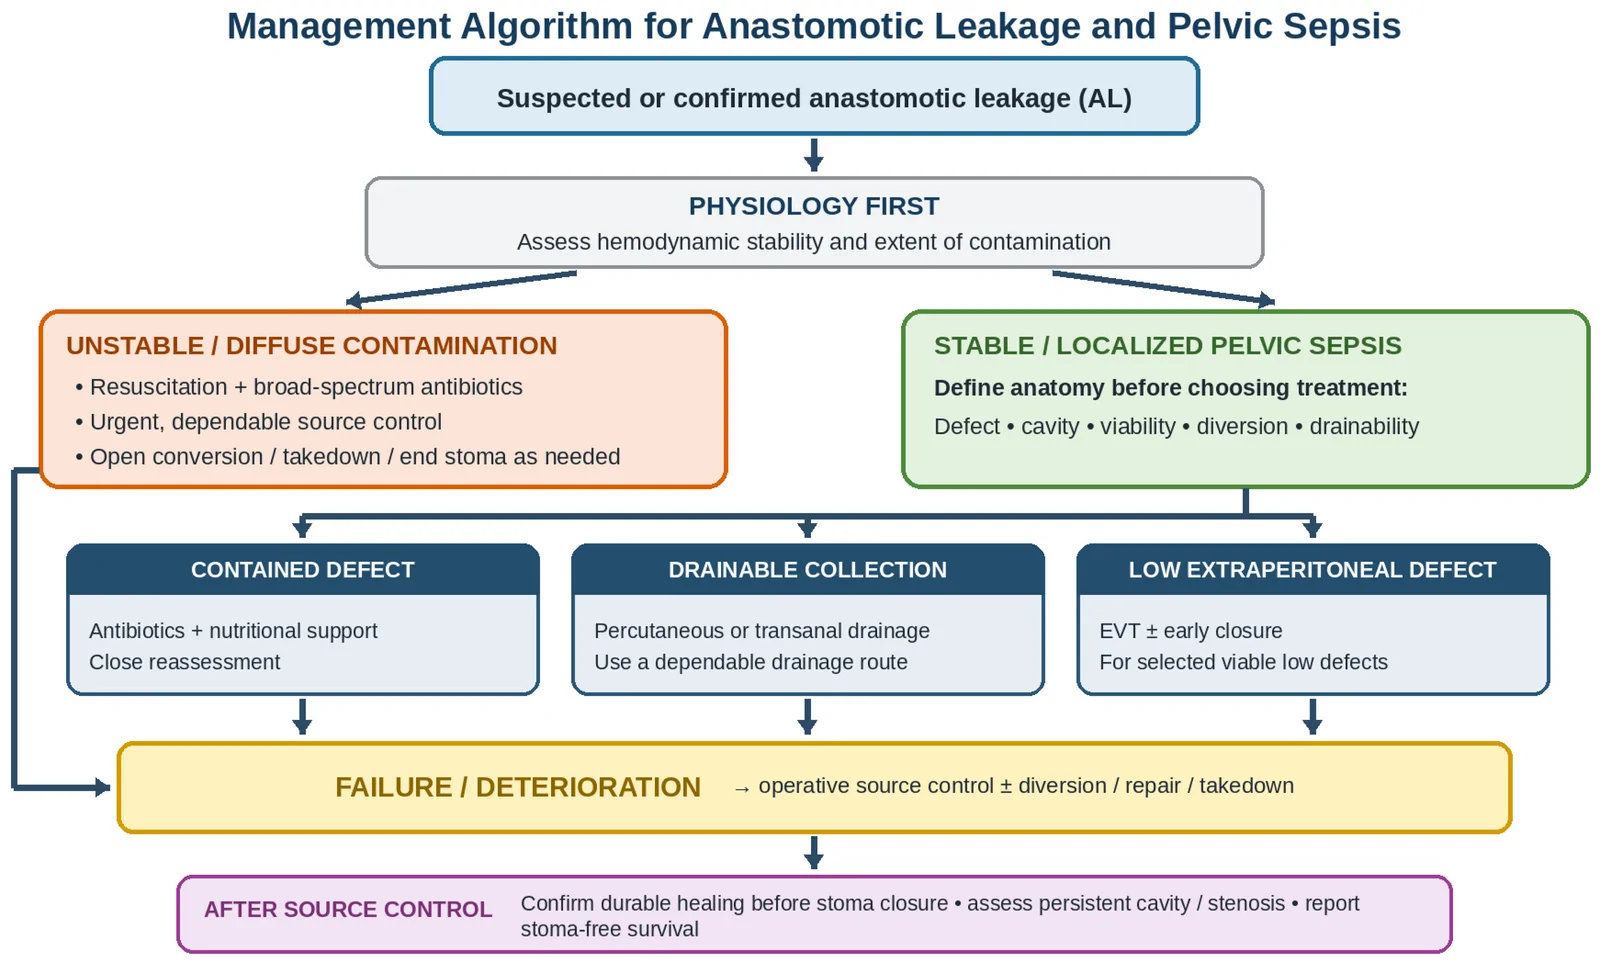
Clinical management algorithm for AL and pelvic sepsis. Physiological instability or diffuse contamination mandates resuscitation and prompt operative source control. In stable localized sepsis, anatomy guides treatment: selected small contained leaks without a drainable abscess may receive antibiotics and nutritional support with close reassessment (pooled success approximately 44% [60]); accessible collections may undergo percutaneous or transanal drainage (approximately 74% [60]); and selected low extraperitoneal defects in viable bowel may undergo EVT with or without early closure (EVT healing approximately 85% [61]). Clips, sutures, and glue are reserved for small viable defects after adequate drainage. Persistent fever or organ dysfunction, feculent output, an enlarging or non-collapsing cavity, or inability to advance nutrition should prompt escalation; reported pooled success is approximately 70% for salvage of a viable anastomosis [60] and approximately 77% after anastomotic takedown [60]. These pooled estimates are heterogeneous and are not head-to-head comparisons. Abbreviations: AL, anastomotic leakage; CT, computed tomography; EVT, endoluminal vacuum therapy. The colors distinguish management phases and decision points rather than quantitative risk levels.
